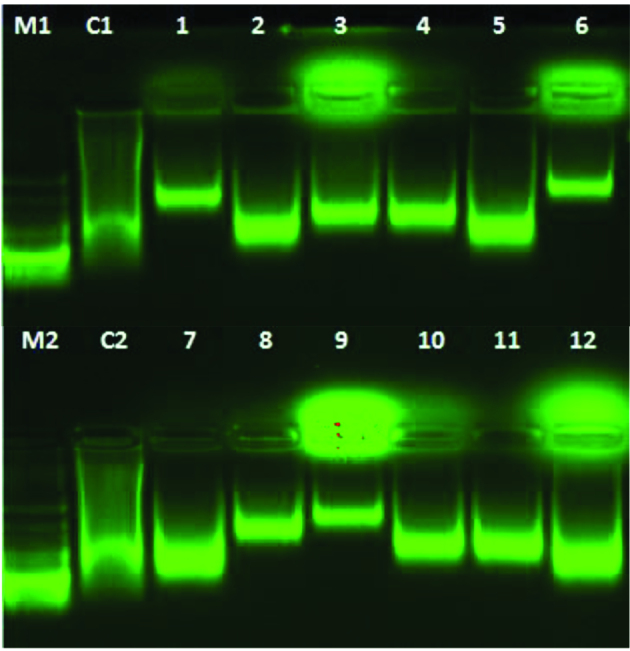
DNA binding of newly synthesized compounds. Lane M1 and M2, DNA Marker; Lane C1 and C2, Control, CT- DNA; Lane 1, CT- DNA + 500 μg/mL of A1; Lane 2, CT- DNA + 500 μg/mL of A2; Lane 3, CT- DNA + 500 μg/mL of A3; Lane 4, CT- DNA + 500 μg/mL of A4; Lane 5, CT- DNA + 500 μg/mL of A5; Lane 6, CT- DNA + 500 μg/mL of A6; Lane 7, CT- DNA + 500 μg/mL of P1; Lane 8, CT- DNA + 500 μg/mL of P2; Lane 9, CT- DNA + 500 μg/mL of P3; Lane 10, CT- DNA + 500 μg/mL of P4; Lane 11, CT- DNA + 500 μg/mL of P5; Lane 12, CTDNA + 500 μg/mL of P6.
